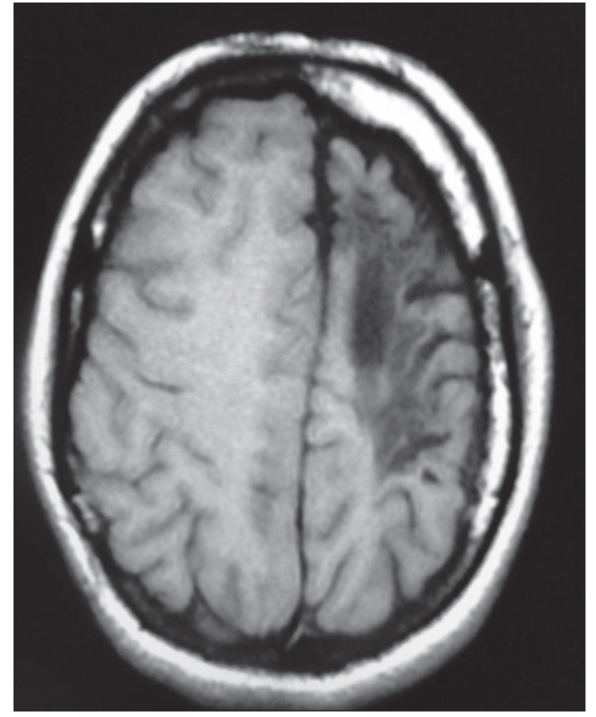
Axial T1-weighted image: Left cerebral hemiatrophy with calvarial thickening. Hypointensity in white matter represents gliosis
